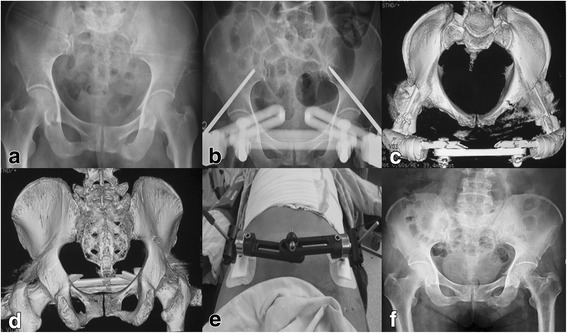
A 60-year-old female patient with anterior pelvic ring fracture due to a traffic accident. a Preoperative X-ray film showing the anterior pelvic ring fracture. b–d Postoperative X-ray film and 3-D CT showing the satisfactory reduction with anterior pelvic external fixation (APEF). e The postoperative incision. f X-ray film showing the healed fracture at postoperative 7 months
